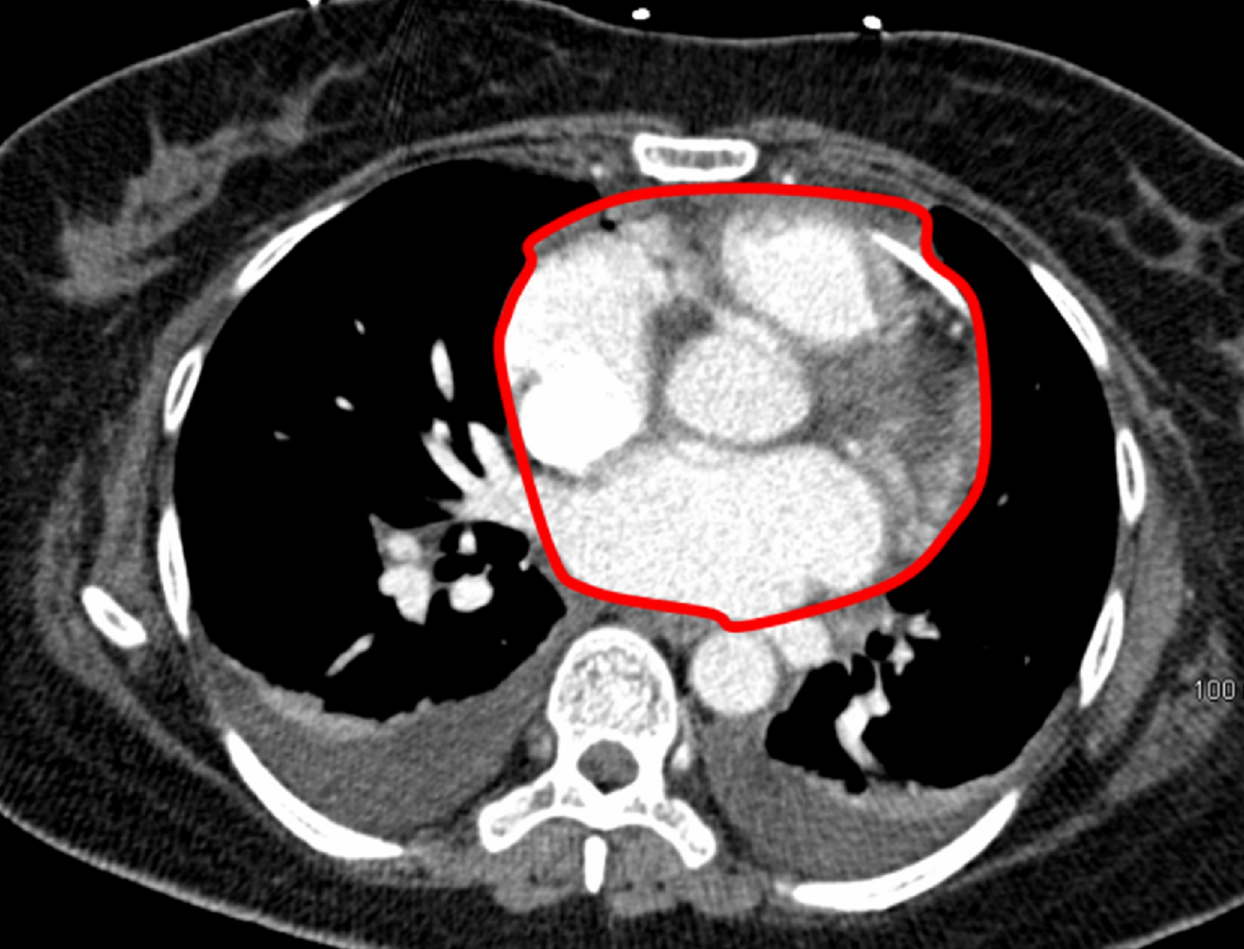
Post-procedural Follow-up Computed Tomography Angiogram of the Chest A 48-hour post-procedural follow-up axial computed tomography angiogram of the chest shows resolution of cardiac tamponade. The computed tomography angiogram shows the lack of blood within the pericardial space, with a homeostatic heart border (red outline).
